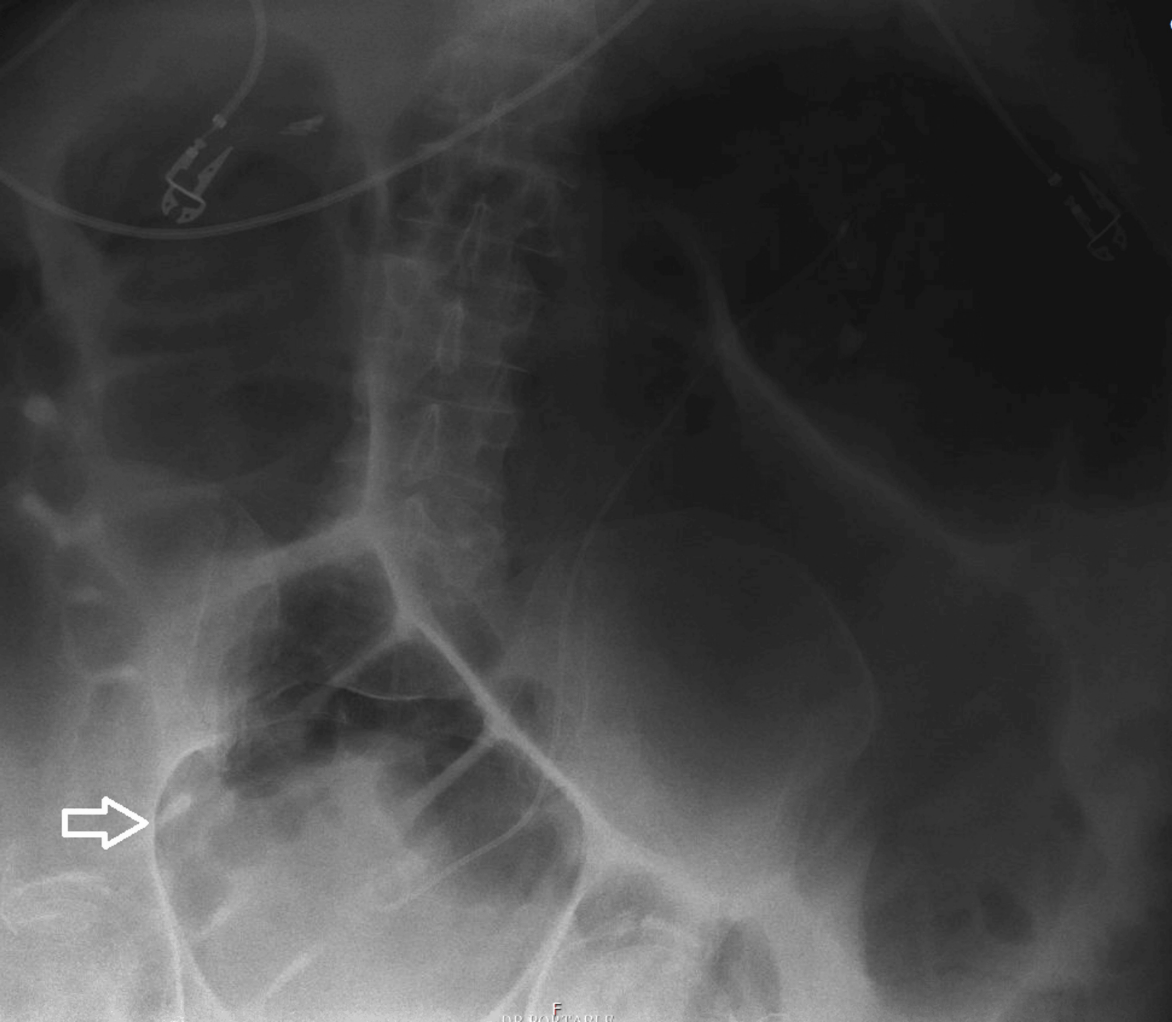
An abdominal X-ray obtained on day 26 of hospitalization showing continued colonic dilatation (arrow)
